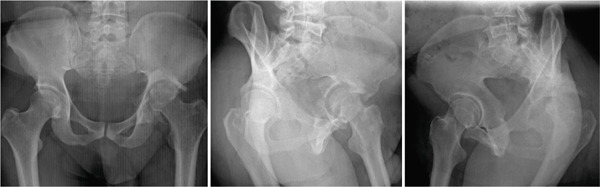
Case 3 preoperative radiographs.
